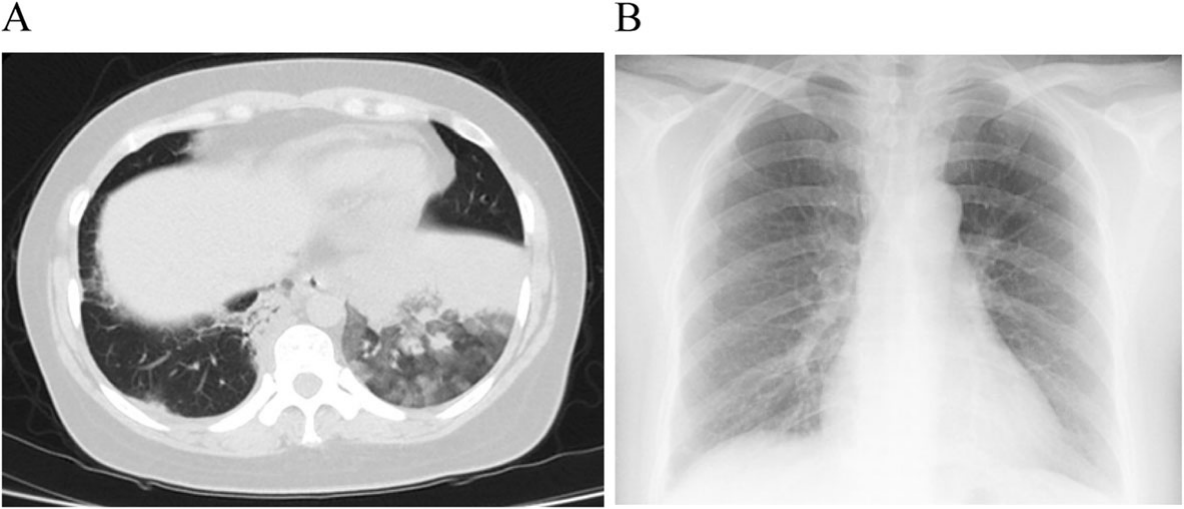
Chest images after the second BT session. a chest CT showed new infiltrates in her left lower lobe after the left lower lobe BT treatment. b Chest X-ray showed the infiltrates in the left lower lobe had disappeared after five-day of antibacterial treatment
